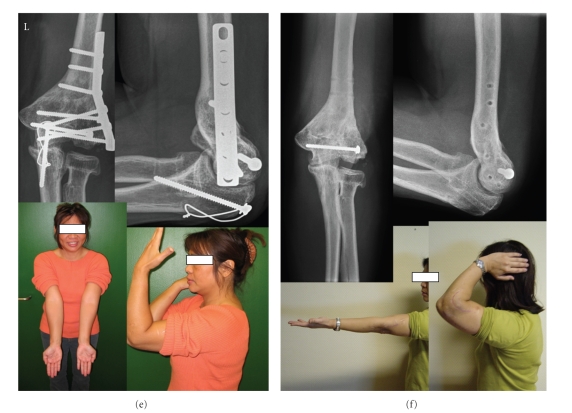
(a) Our patient was a 48-year-old dentist who was seen 10 months after a high energy fall that resulted in a complex intra-articular fracture of her left nondominant distal humerus. (b) Initial treatment performed at an outside institution consisted of static external fixation with the arm in 90° flexion and the forearm in neutral rotation. (c) The external fixator was removed after 8 weeks and follow-up the radiographs revealed distal humerus intra-articular malunion. (d) An oscillating saw was used to create a trapezoid wedge to correct overlength varus of the lateral column and flexion of the capitellum in order to realign capitellum and trochlea. Extreme caution was taken no to disrupt blood supply of the capitellum. (e) The patient had loss of anterior translation of the distal end of the humerus resulting in loss of flexion of 35 degrees, with 10 degrees of hyperextension of the left elbow. (f) Patient opted for removal of hardware and extra-articular excavation of the prominent ventral distal humerus creating a new fossa coronoidea in order to gain flexion.
